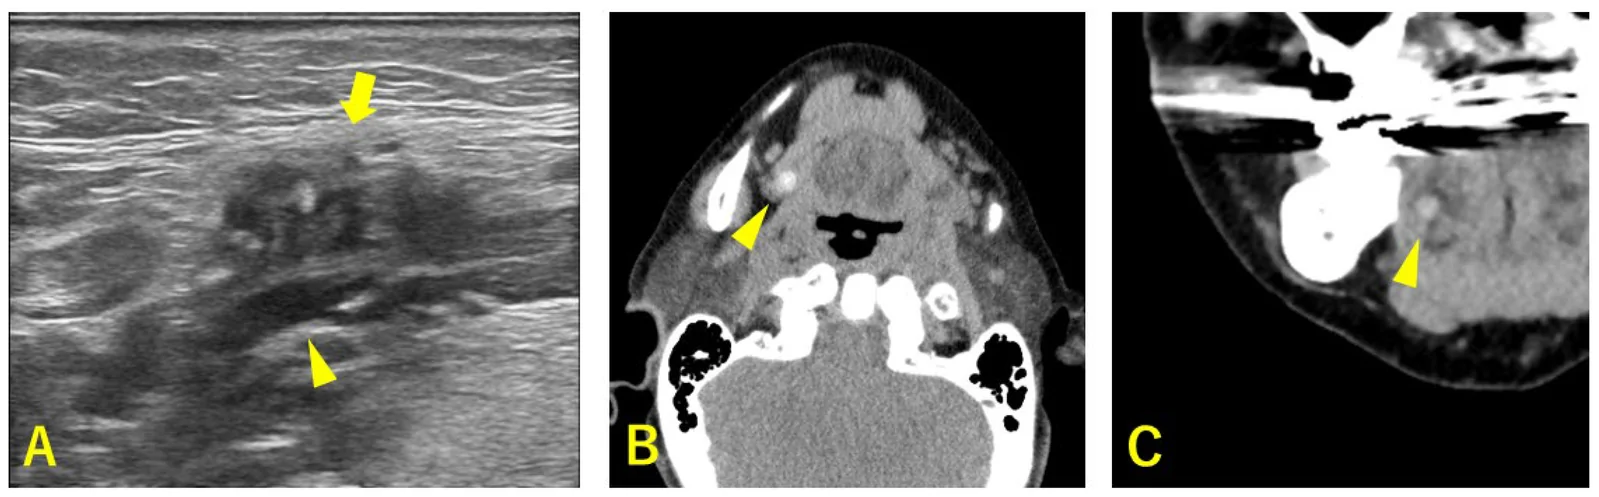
Computed tomography (CT) and ultrasonography findings after right submandibular gland excision: (A) Ultrasonography showed no residual calculi (arrow) and persistent dilatation of Wharton’s duct (arrowhead). (B,C) The high-attenuation area within the right Wharton’s duct, with a mean CT value of 159 HU, and the surrounding edematous changes were slightly reduced but remained present (arrowheads).
